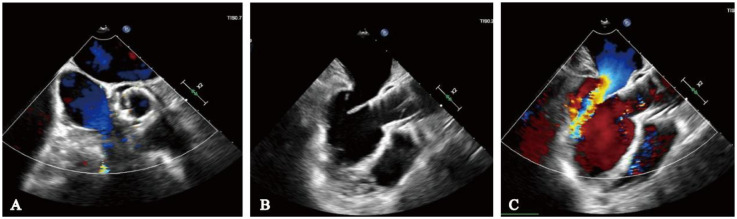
Intraoperative transesophageal echocardiography monitoring. (A) No paravalvular leak on short-axis view; (B) long-axis view indicates adequate valve depth, no impairment of anterior mitral leaflet function; (C) ultrasound color Doppler shows no aortic regurgitation.
